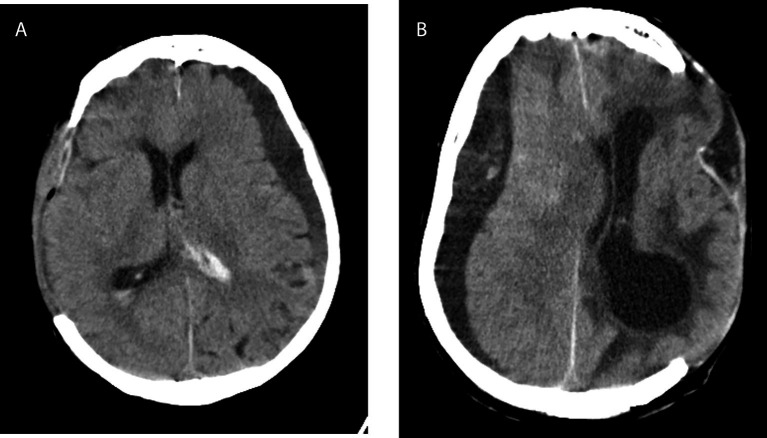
Check-up axial brain CT scan on day 9 post-injury showing a regressive dynamic of intracranial hemorrhage and brain edema, but also the contralateral formation of subdural hygroma producing a midline shift to the right for 7 mm (A). Check-up axial brain CT of another patient on day 15 post-injury, depicting a peri-hemispheric right-sided subdural hygroma with a mass effect and midline shift, contralateral to the evacuated left-sided aSDH and accompanied by the right lateral ventricle compression, as well as dilatation and displacement of the left lateral ventricle, whose temporal horn is extremely enlarged (B).
